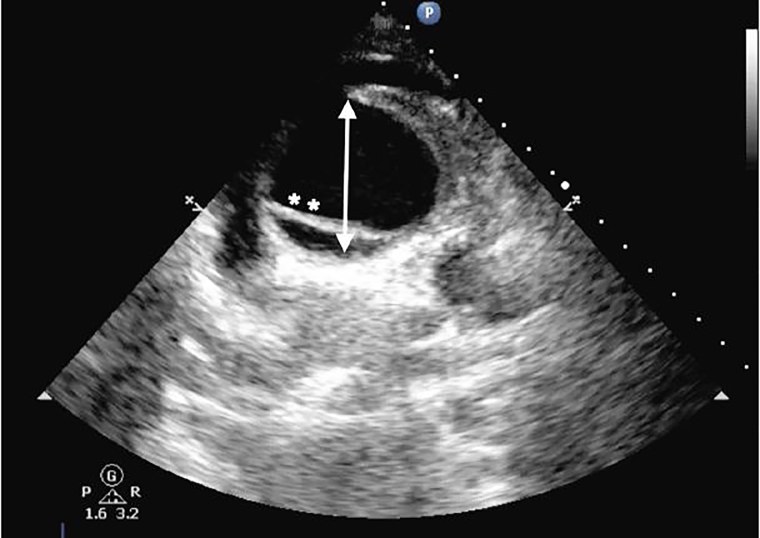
Suprasternal notch short-axis view performed on point-of-care ultrasound in elderly patient presenting to the emergency department after a syncopal event; aortic arch (arrow) with dissection flap (stars).
